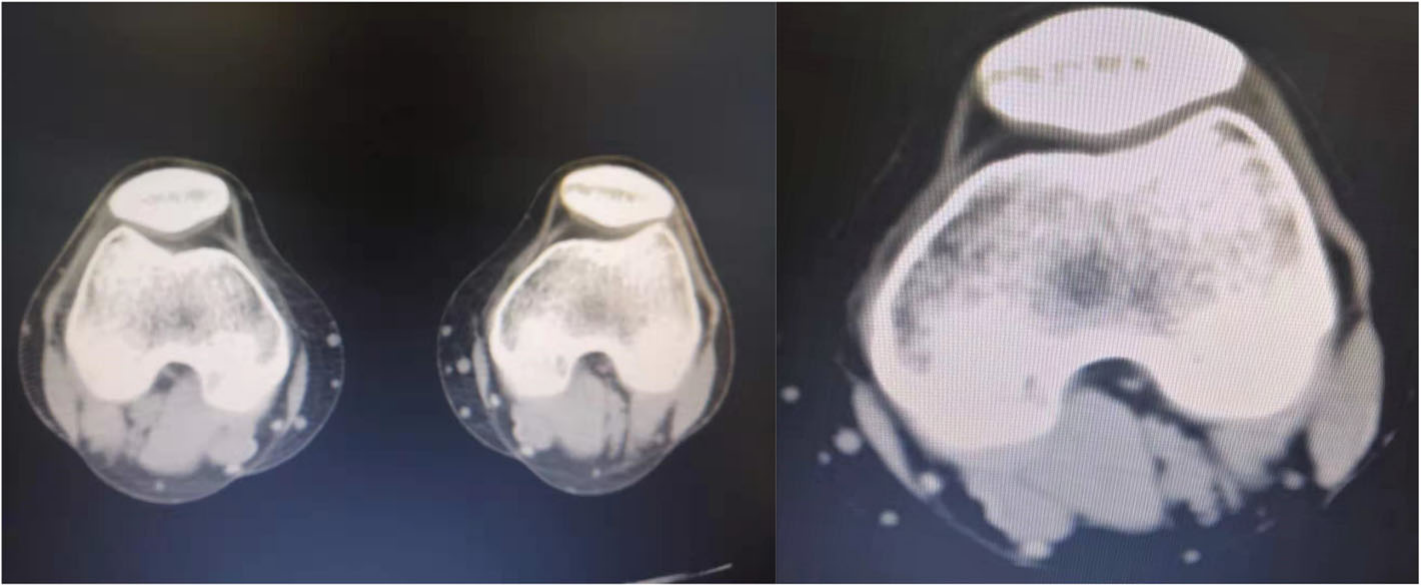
A 22-year-old woman with bilateral knee pain (bilateral CT images) for more than 10 years. Postoperative CT images show significant improvement
